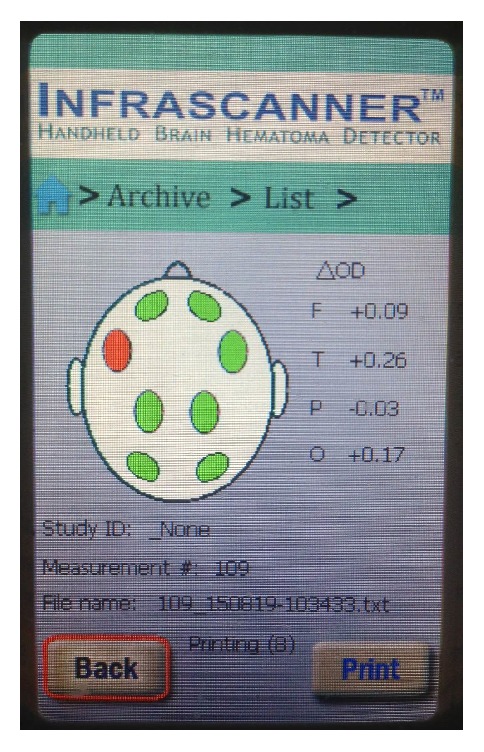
Example of an Infrascanner measurement of a sedated, intubated motor-cross rider in the HEMS setting. This prehospital measurement suggested an intracranial hematoma in the left parietal region (red dot), which was later confirmed by the in-hospital CT scan.
